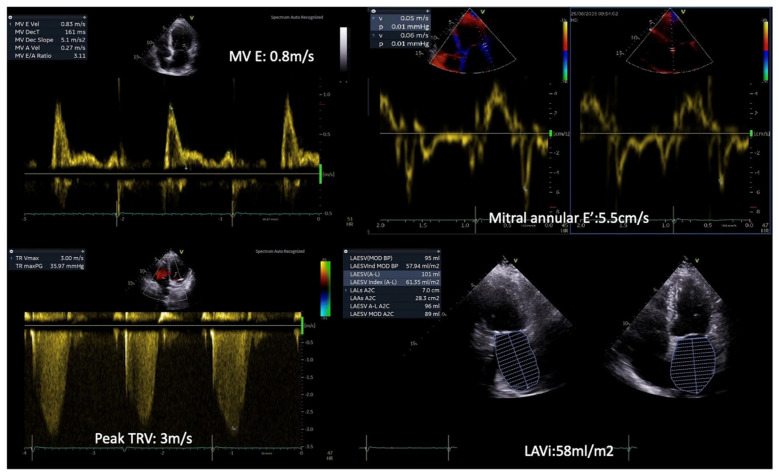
Patient with T2DM and exertional dyspnea. Rest echocardiography revealed diastolic dysfunction grade III, with an MV E/E’ ratio = 15, a Peak TR velocity: 3 m/s and severe left atrial dilatation (LAVi: 58 mL/m^2^). (Original images from authors’ institutions).
